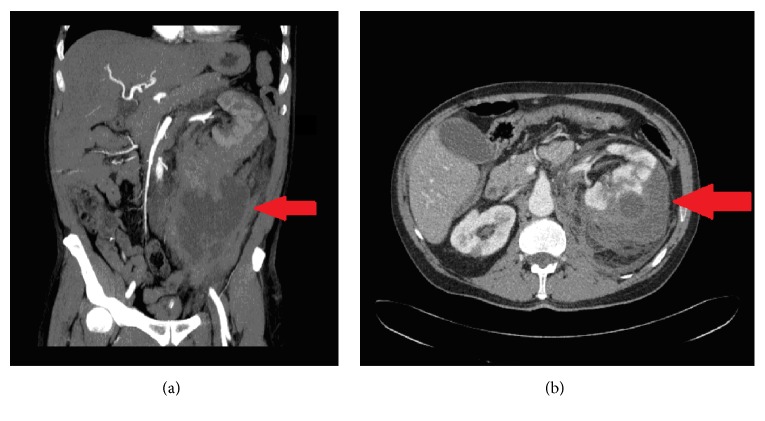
A coronary and a horizontal view of an abdominal CT with intravenous contrast that revealed a 17x11x8cm^3^ spontaneous retroperitoneal hematoma (shown with arrow) due to bleeding of an intraparenchymal branch of the left renal artery extending from the left kidney down to the pelvis are shown ((a) and (b), respectively).
